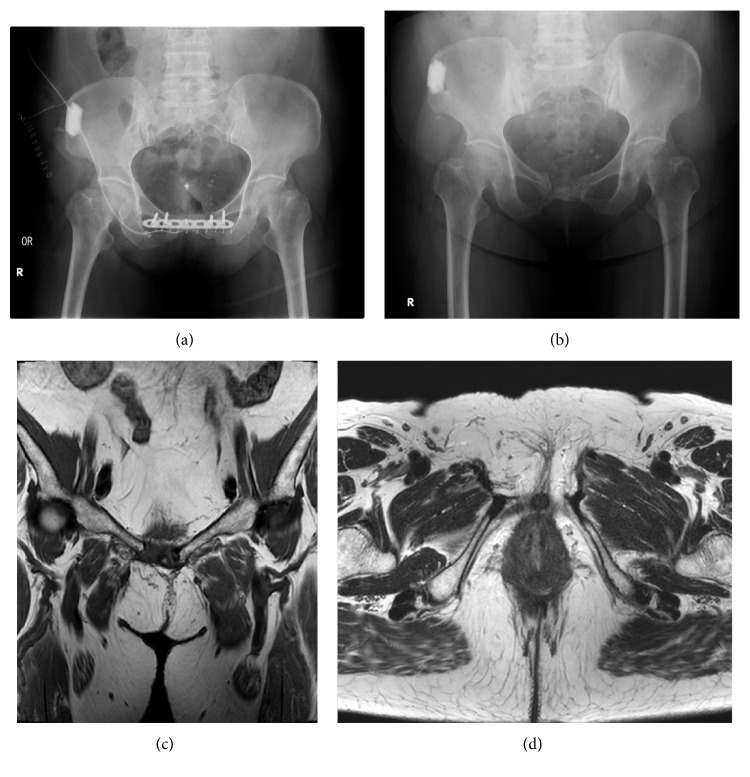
Postoperative standing radiographs (anterior-posterior view) of the pelvis and hips, obtained immediately after surgery (a) and at 4 years postoperatively (b). (c) and (d) Magnetic resonance images obtained at 4 years postoperatively. There is no evidence of tumor recurrence.
